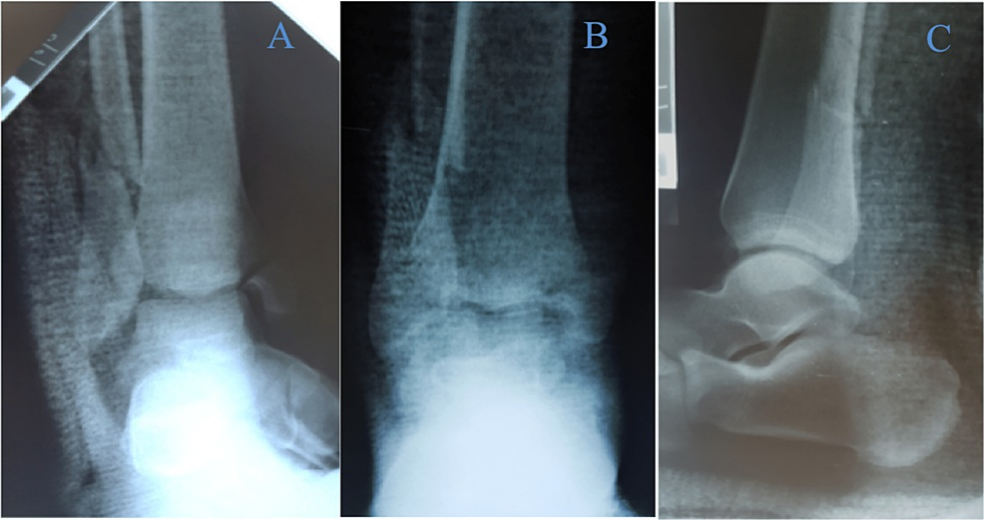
Plain radiographs post-reduction (mortise, AP and lateral views) A - mortise view, B - AP view, C - lateral view AP: anteroposterior
